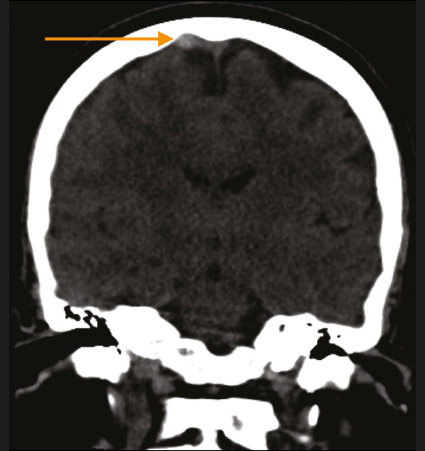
Coronal reconstruction of an unenhanced CT image showing
